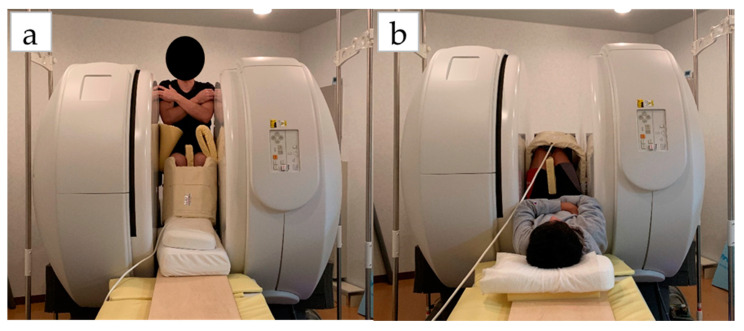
Measurement of position on vertical MRI: (a) sitting position; (b) lying position.
